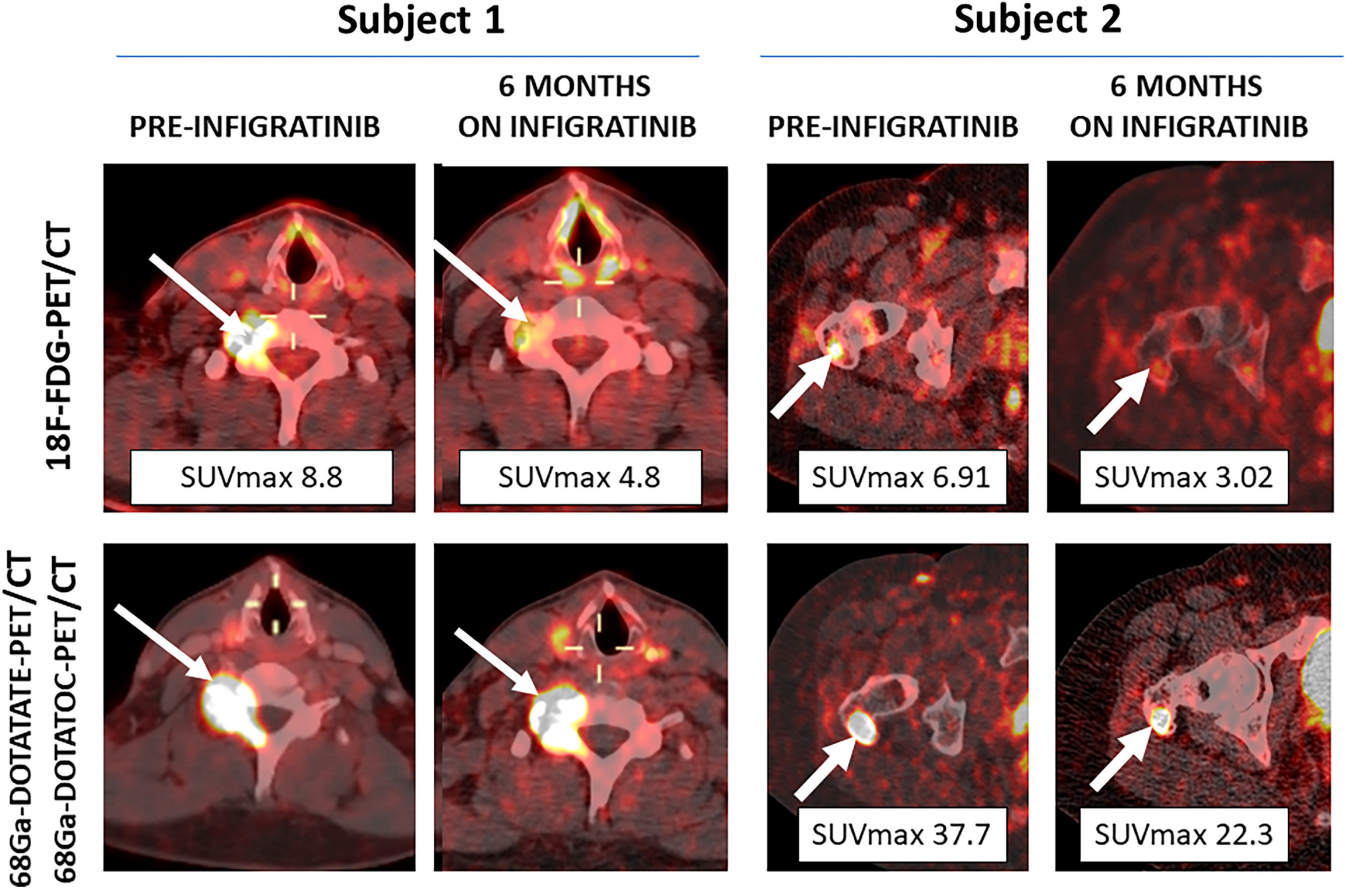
18F‐FDG‐PET/CT, 68Ga‐DOTATATE‐PET/CT, and 68Ga‐DOTATOC‐PET/CT scans from subjects 1 and 2 prior to infigratinib initiation and after 6 months of therapy. Phosphaturic mesenchymal tumors of the C7 vertebra (subject 1) and right greater trochanter (subject 2) are indicated by white arrows. Subject 1 had a pre‐infigratinib 68Ga‐DOTATOC‐PET/CT and post‐infigratinib 68Ga‐DOTATATE‐PET/CT, limiting quantitative comparison of the lesions, although there was a subjective decrease in uptake. In all other studies, the SUVmax of the PMTs were reduced by greater than 40% after treatment, without appreciable change in size. SUVmax = maximum standardized uptake value.
